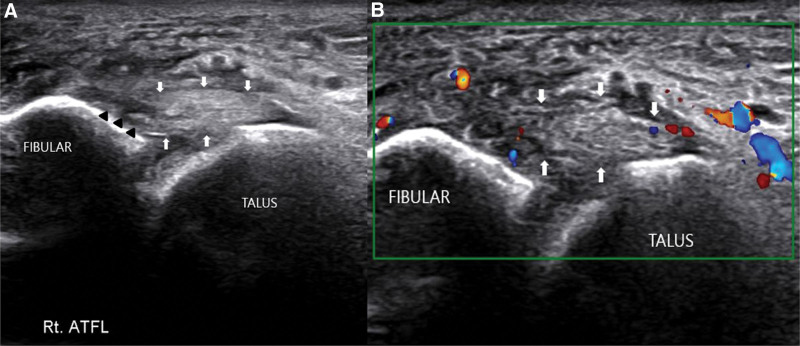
Ultrasonography of right ATFL (arrow). (A) Partial tear of anterior right ATFL (arrowhead). (B) Increased vascularity around right ATFL on doppler image. ATFL = anterior talofibular ligament.
